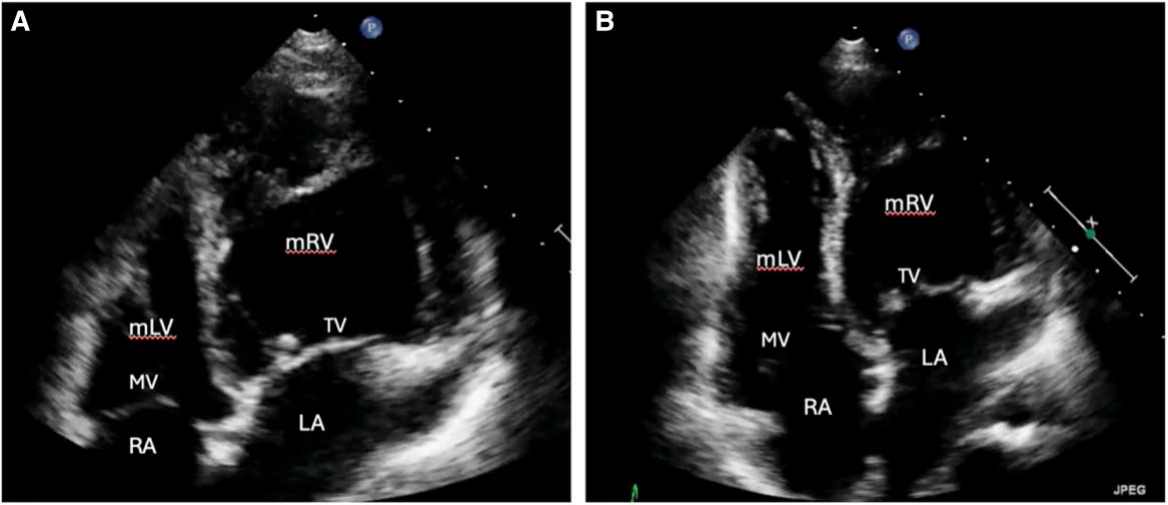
Transthoracic echocardiographic findings of a 42-year-old woman after OHCA. The right ventricle shows predominant apical trabeculation and the presence of a moderator band *(A)*. Additionally, the apically displaced tricuspid AV valve is displayed *(B)*. LA, left atrium; mLV, morphologic left ventricle; mRV, morphologic right ventricle; MV, mitral valve; RA, right atrium; TV, tricuspid systemic AV valve.
